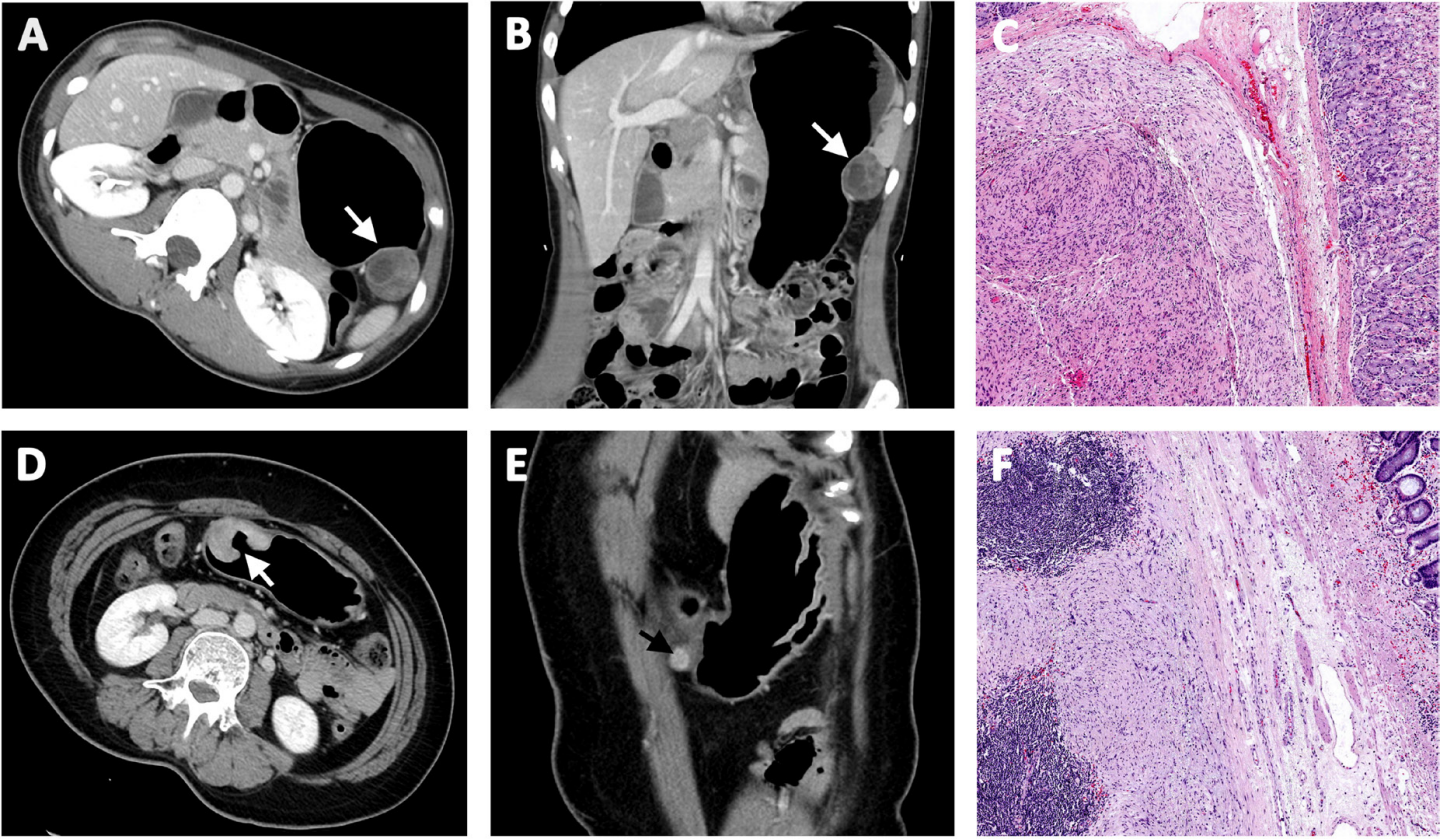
Representative preoperative computed tomographic image of gastric schwannoma (A) without regional lymphadenopathy (B) and corresponding pathologic findings without lymphoid cuff (C). A case of gastric schwannoma (D) with regional lymphadenopathy (E) and corresponding pathologic findings with lymphoid cuff (F).
